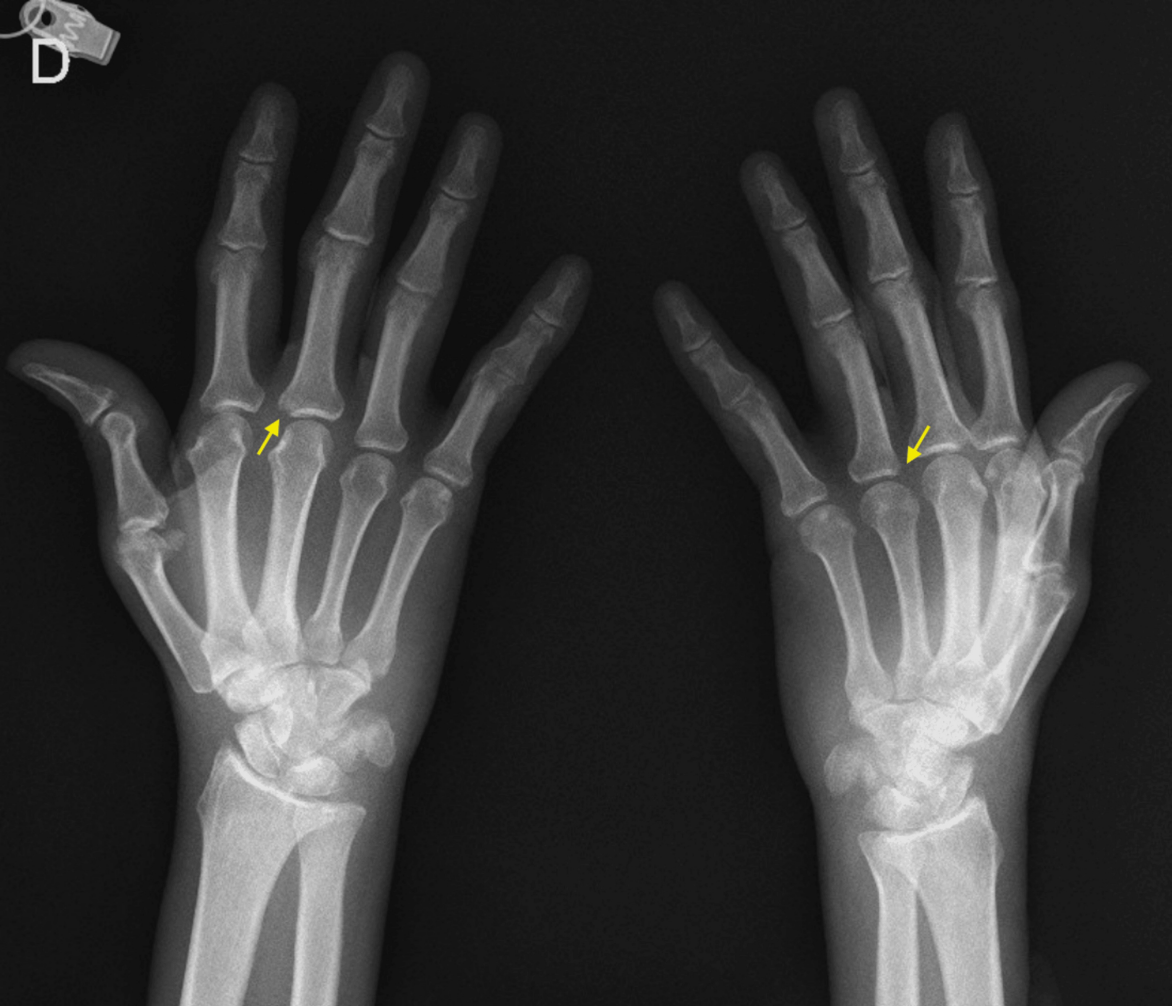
Hand X-ray Multiple marginal erosions as indicated by the yellow arrows
